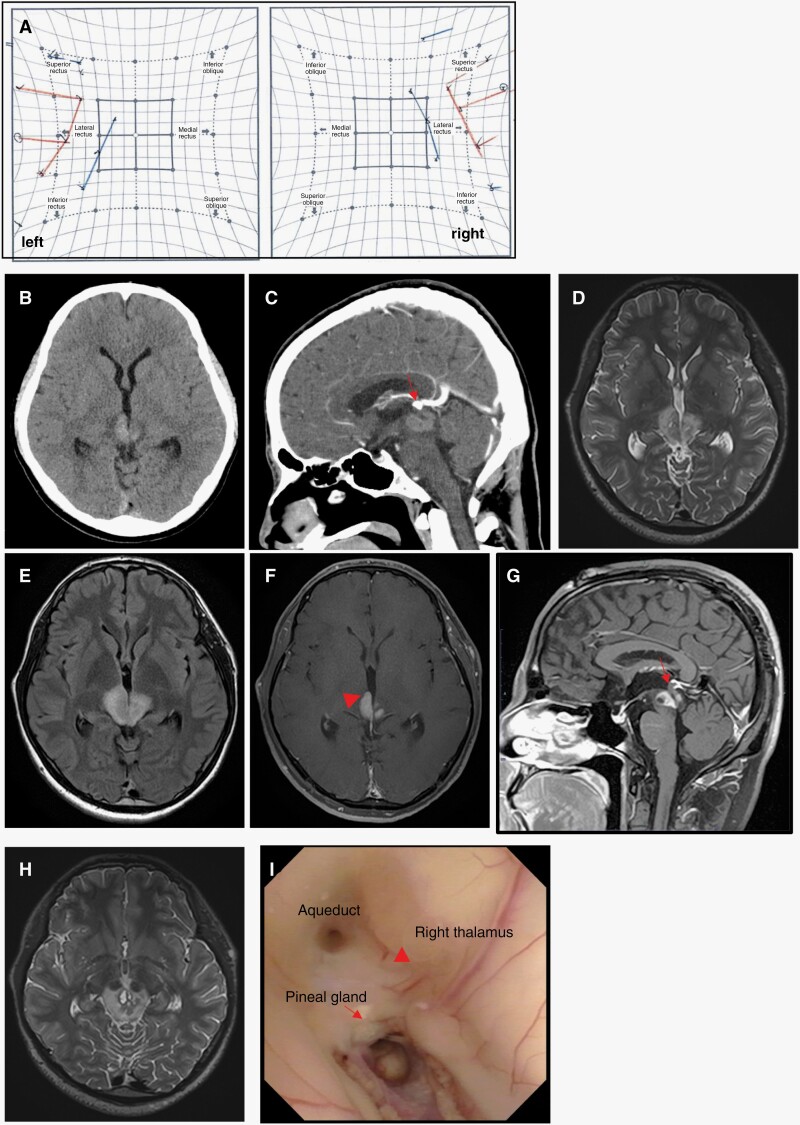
Preoperative and intraoperative findings. (A) Preoperative Hess screen test. (B, C) Computed tomography imaging (axial [B], sagittal [C]) showing high-density tumor at the dorsal midbrain. The pineal region with calcification is located at the upper edge of the tumor (arrow). (D, E) T2-weighted (D) and fluid-attenuated inversion recovery (E) magnetic resonance images showing iso- to slightly high-intensity lesion surrounded by hyperintense area. (F) Gadolinium-enhanced T1-weighted imaging demonstrating midbrain tumor extended to the medial of the bilateral thalamus (arrowhead). (G) Intact pineal gland (arrow). (H) T2-weighted imaging showing cystic lesions. (I) Operative findings. Right thalamic lesion (arrowhead) and calcified pineal region (arrow).
